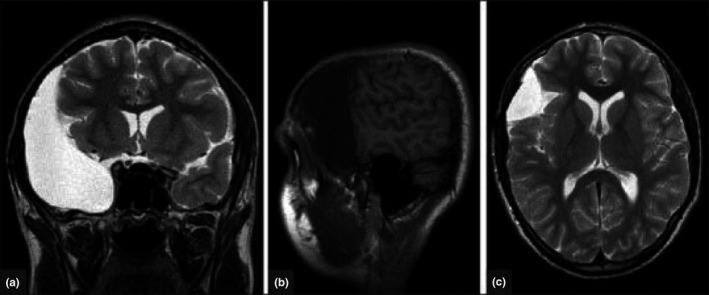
MRI examination of bladder fenestration for treatment of intracranial arachnoid cysts
